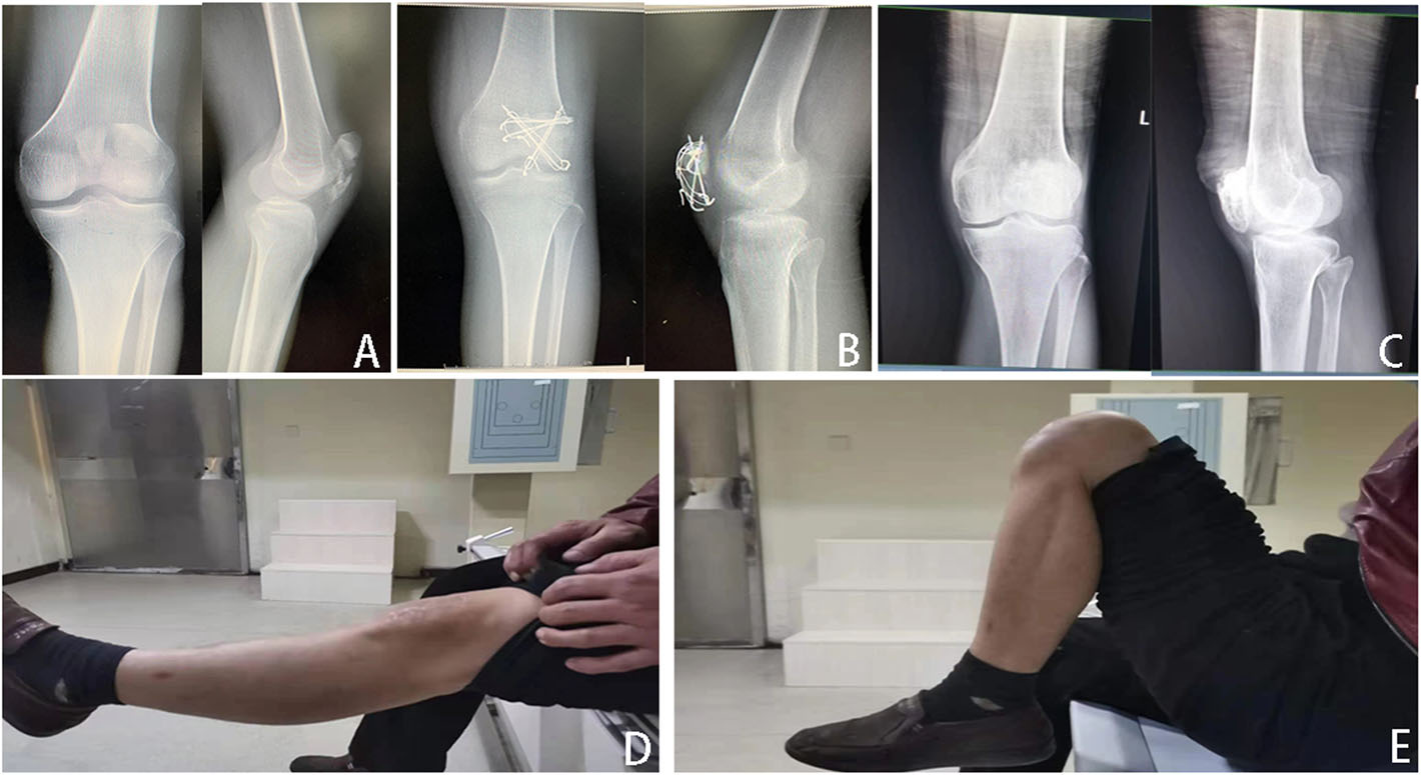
A Male, 41 years old, traffic accident, anteroposterior and lateral radiographs of the left knee showed patellar comminuted fractures preoperatively and B postoperatively at 1 year showed the fracture was well healed. C Anteroposterior and lateral radiographs of the left knee postoperatively at the final follow-up showed patellofemoral arthritis. D Range of motion was restored normally at the final follow-up
